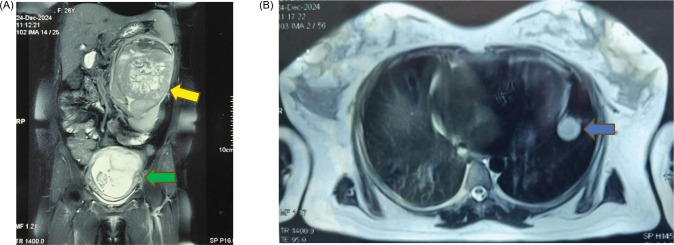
(A) T2 weighted Magnetic Resonance Imaging (MRI) of Kidney, Ureter and Bladder (KUB) region coronal view showing a large lesion in left suprarenal region causing inferior displacement of left kidney and compressing adjacent structures including pancreas and spleen (Yellow Arrow). Intrauterine gravid uterus was also seen (Green Arrow). (B) T2 weighted MRI of Scanned portion of chest mentioned about suspected lung metastasis (Blue Arrow).
